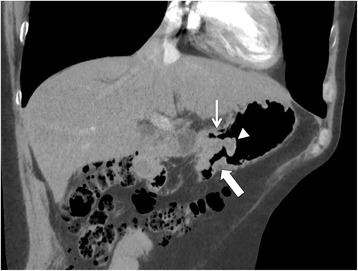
CT scan with contrast injection: coronal view. Both afferent (thin arrow) and efferent (large arrow) jejunal loops are visible close to the gastrojejunostomy site, where the gastric cancer (arrowhead) protrudes into the gastric lumen
